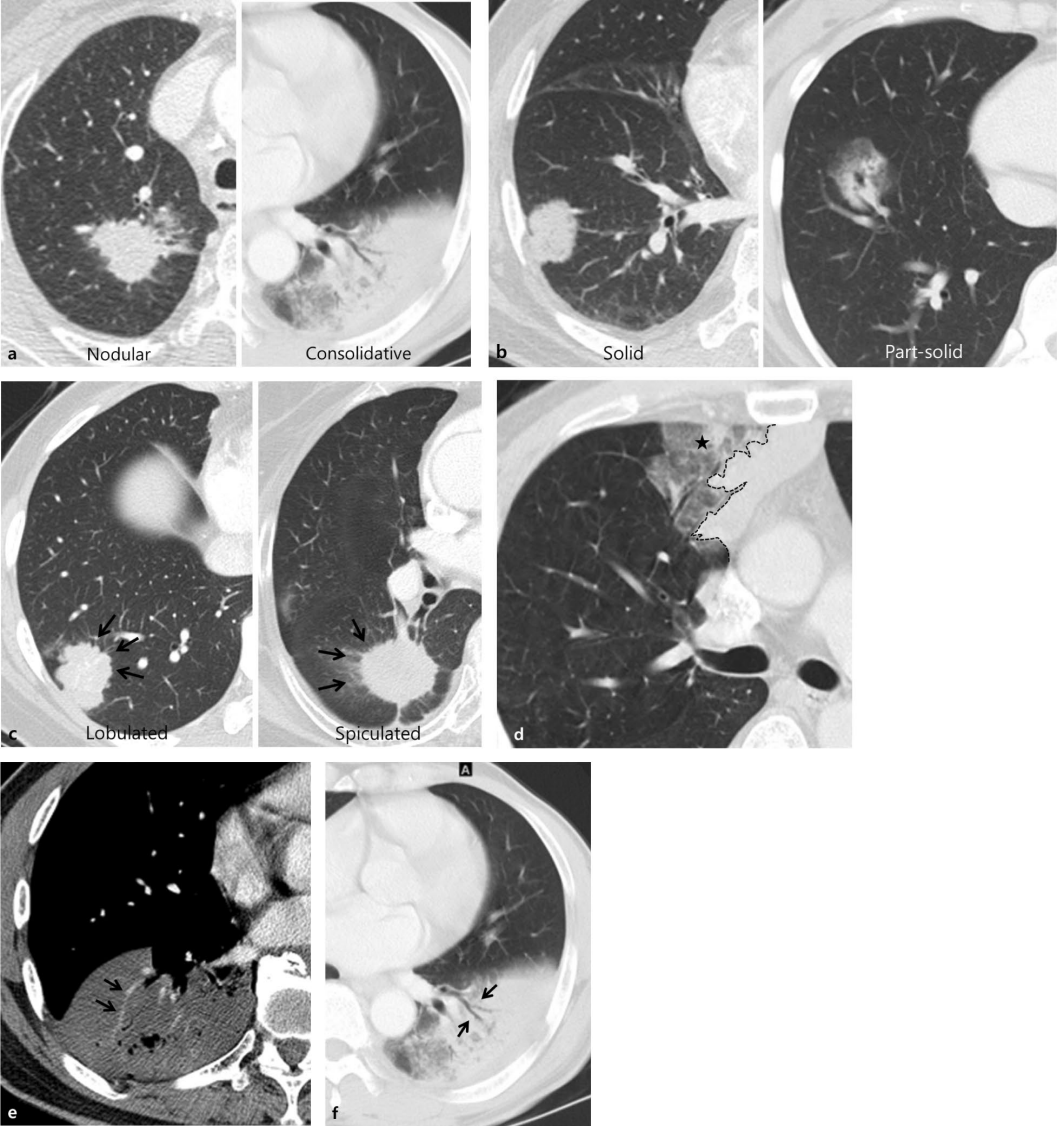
Examples of CT images of morphology (a), solidity (b), margin characteristics (c), well-defined heterogeneous ground-glass opacity (d), CT angiogram sign (e), CT air-bronchogram sign (f) showing typical features of invasive mucinous adenocarcinomas of the lung. Well-defined heterogeneous ground-glass opacity (asterisk) defined as the combination of consolidation and ground-glass opacity detected beyond the imaginary margin of the lesion (dashed line)
